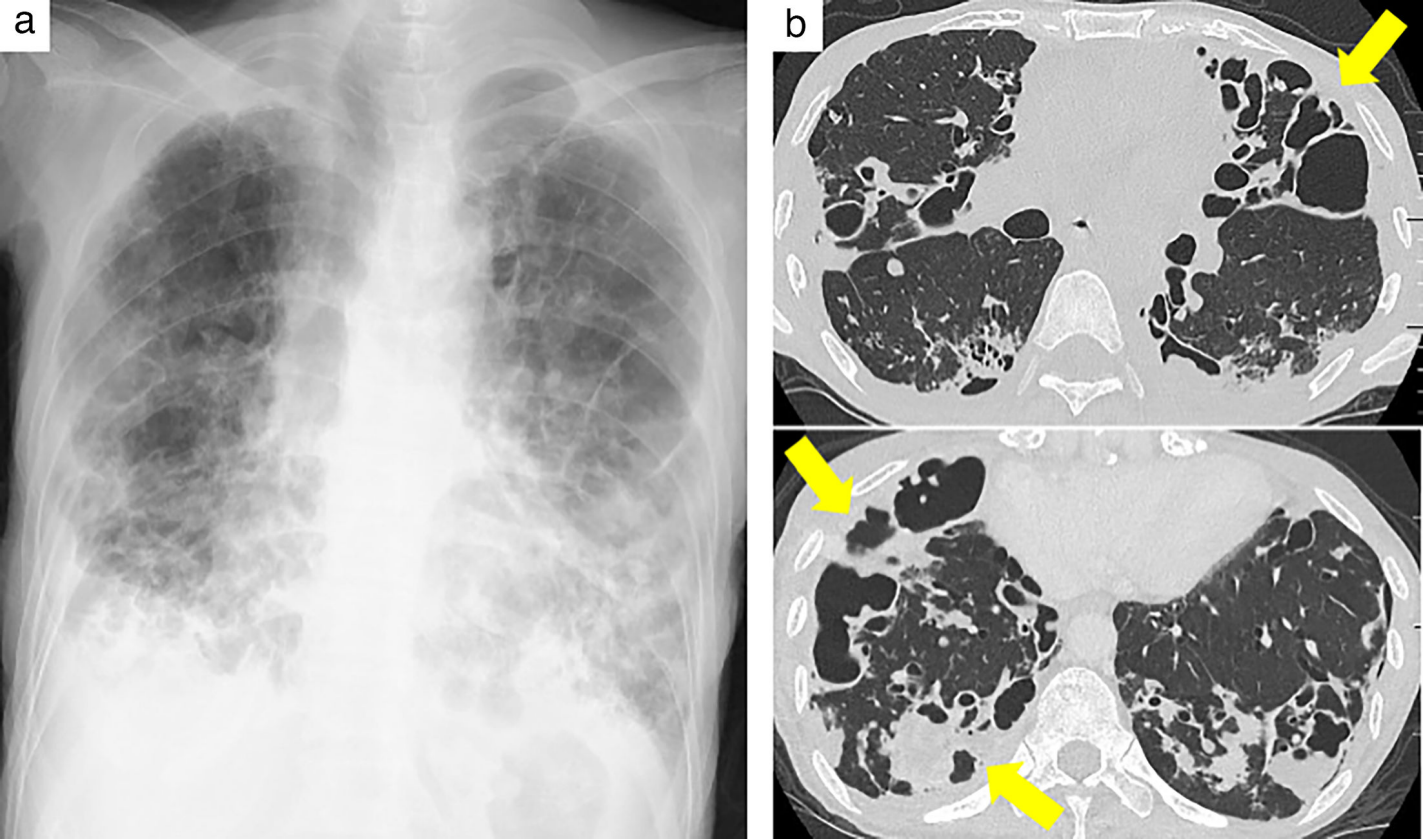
Chest radiography and high-resolution computed tomography (HRCT) on admission. (a) Chest radiograph showing infiltrates, reticulonodular opacities, and cavities in both lungs. (b) HRCT image showing bronchiectasis, multiple nodules, consolidations, pleural thickening, and cavity lesions of various sizes, with a bilateral and multilobular distribution. Findings indicate marked disease progression (arrows).
